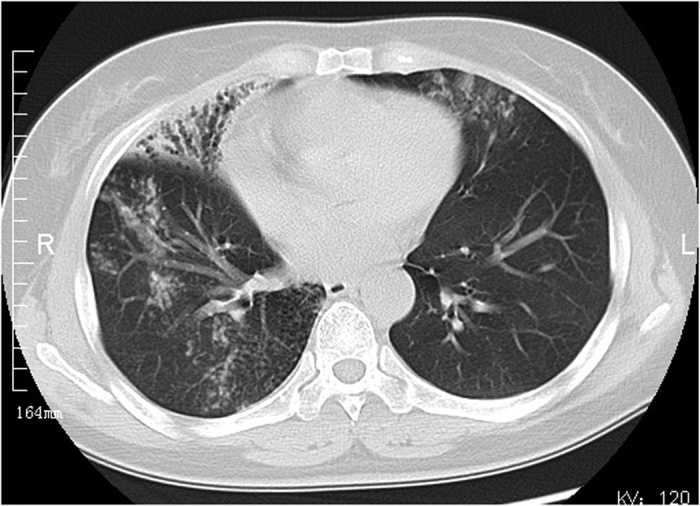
M. intracellulare* lung disease in a 57-year-old woman. Chest CT scan shows centrilobular nodules and bronchiectasis. Also note lesions predominate in lingular segment and right middle lobe.
